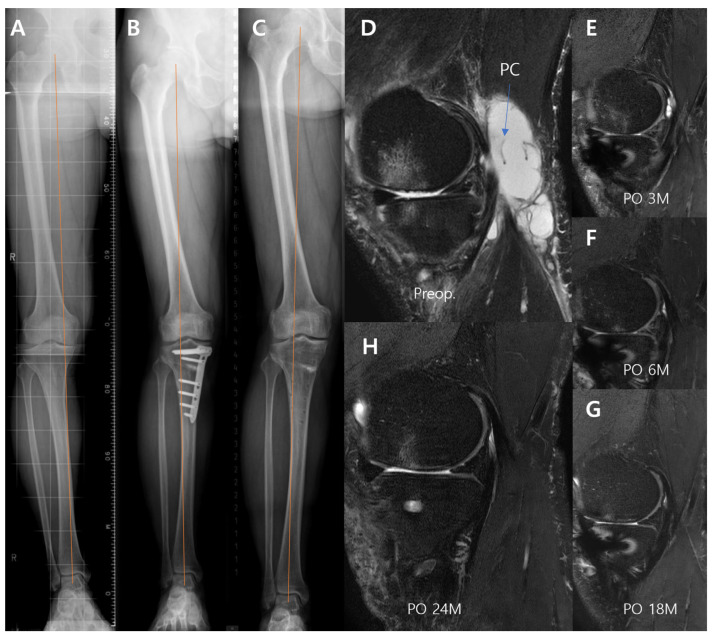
Changes in the simple radiographs and popliteal cyst in MRI after medial open-wedge high tibial osteotomy (MOWHTO): preoperative medial compartment osteoarthritis (OA) with varus alignment (A); right knee of 62-year-old male patient is corrected to valgus alignment via MOWHTO (B); maintained well at postoperative 2 years after plate removal (C); preoperative popliteal cyst (D); cyst decreased with time after MOWHTO, observed through serial MRI follow-up to postoperative 24 months (E–H). PC, popliteal cyst; preop., preoperative; PO, postoperative.
